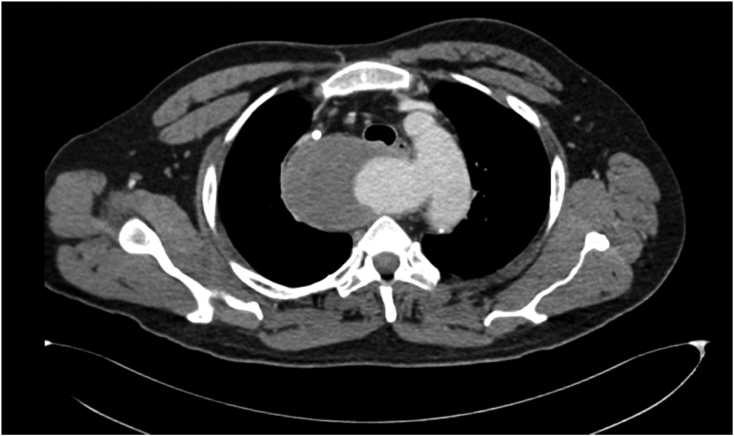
CT scan of the patient showing Kommerell's diverticulum.
